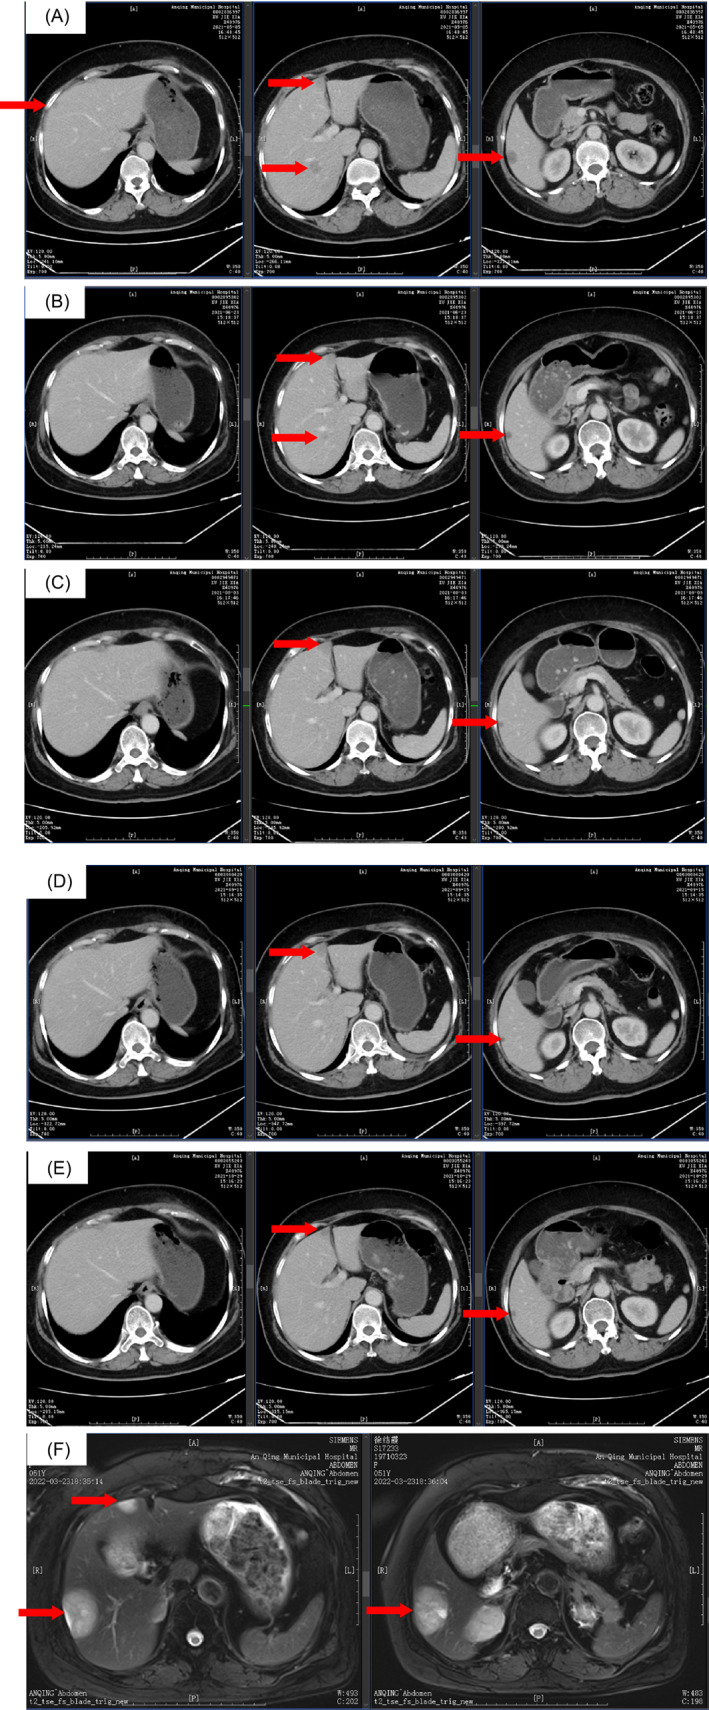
Abdominal CT scan images. (A) Liver metastases prior to the study enrollment (arrow, 2021.05.05 CT). The results revealed low‐density lesions in the right lobe of the liver, with the larger foci about 19 mm in diameter. Cancer metastasis was suspected. (B–D) The abdominal enhanced CT examination at the second, fourth, and sixth cycles of treatment revealed that the tumor continued to shrink (from 12 to 6 mm) and some liver metastases completely disappeared (B: 2021.06.23; C: 2021.08.03; D: 2021.09.15). (E) As the patient was HR positive, the treatment regimen of “Inetetamab + rapamycin + paclitaxel” was changed to “inetetamab + rapamycin + exemestane” for 8 cycles and liver metastases lesion was shrunk to 3 mm (arrow, 2021.10.29 CT). (F) The abdominal MRI showed an increase in the volume of intrahepatic multiple metastatic tumor lesions, with the largest lesion size about 0.5 cm × 3.3 cm, and an abnormal signal in the right adrenal gland (arrows, 2022.03.23 MRI). [Correction added on 5 September 2023, after first online publication: In Figure 2, the positioning of the arrows has been corrected in this version.]
